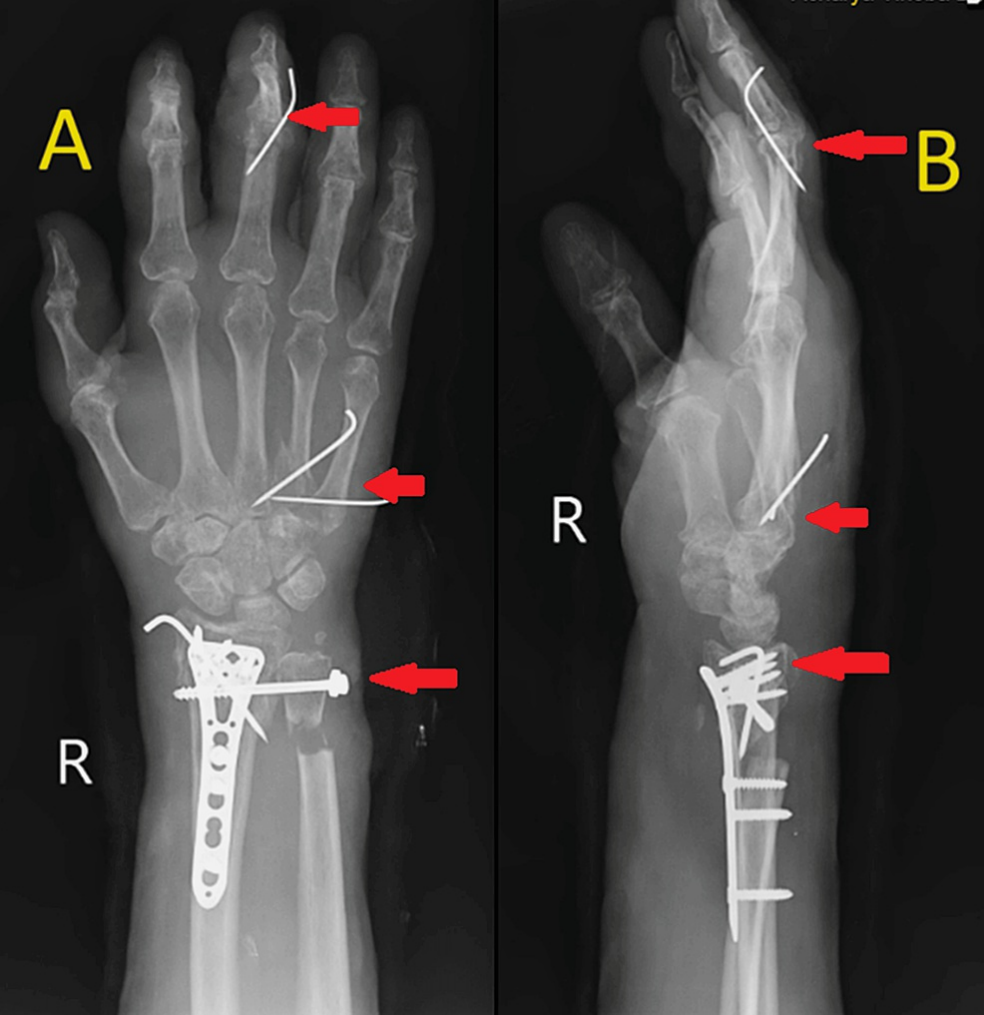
Postoperative X-ray of the right wrist with hand anteroposterior (A) and lateral (B) views (A, B) show open reduction and internal fixation with plate osteosynthesis and bone grafting for the malunited distal end radius fracture and cannulated cancellous (CC) screw fixation for the distal radioulnar joint disruption.
